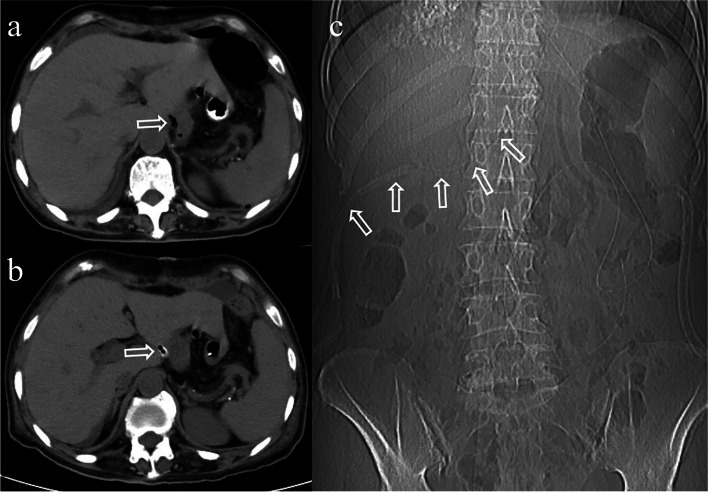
Computed tomography is performed to confirm the tube’s position. Photograph (a) showed the front end of the surgical drainage tube under the esophagojejunal anastomosis. b Showed the triple-cavity tube takes the place of the surgical drainage tube under the esophagojejunal anastomosis, which is precise placed by guide wire exchange method. c Showed the path of the triple-cavity tube
